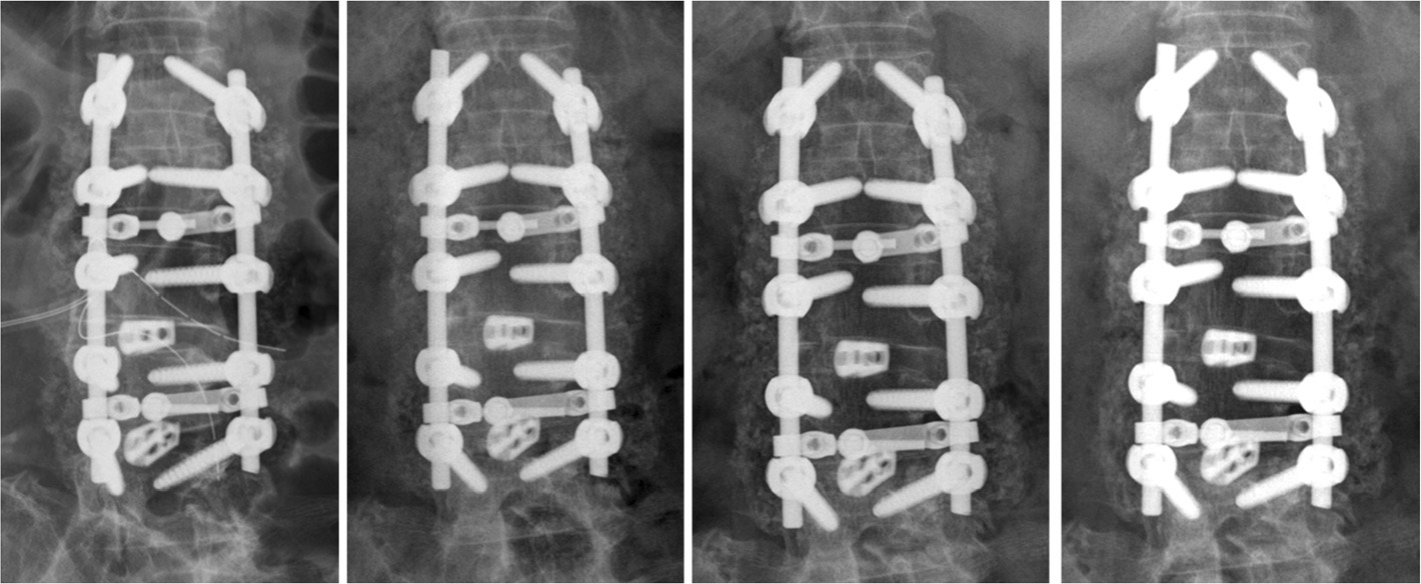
Radiographs from left to right showed the immediately, 3, 6, and 12 months postoperatively. The immediately postoperative image showed multiple opacity spots at the intertransverse space indicated a mixture of hydroxyapatite-β-tricalcium phosphate granules with laminectomy bone chips and DBM. The 12-month image showed a successful fusion mass with continuously qualitative bony bridging at the intertransverse fusion areas
